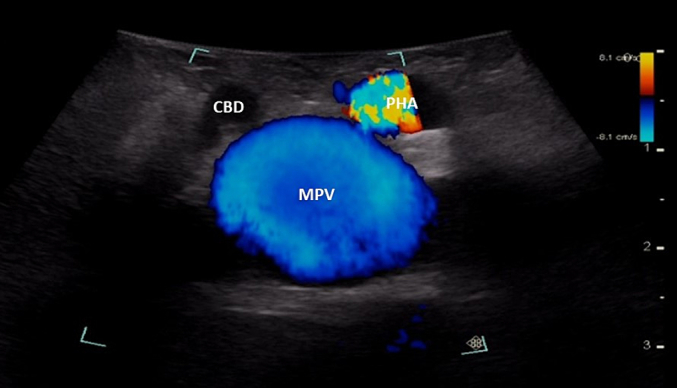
A lack of blood flow on Doppler ultrasound differentiates the bile duct from the hepatic artery and portal vein. MPV main portal vein, CBD common bile duct, PHA proper hepatic artery.
